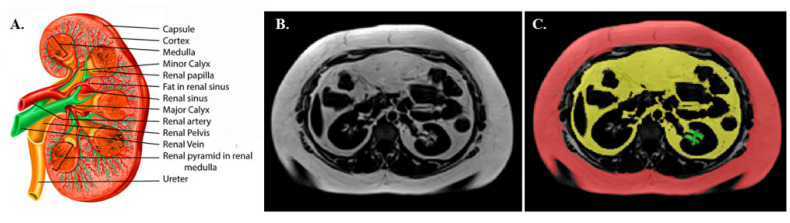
Sketch of the renal anatomy (Source: [29] distributed under the terms of the Creative Commons Attribution 4.0 International License (http://creativecommons.org/licenses/by/4.0/, accessed on the 6 May 2022) © 2022, StatPearls Publishing LLC, (A). Representative examples of an MRI (B) and RSF measurement (green), SAT (red) and VAT (yellow) volume (C). For RSF, a single MRI slice approach was followed. RSF was manually traced, excluding the renal artery and vein from the RSF measurements.
